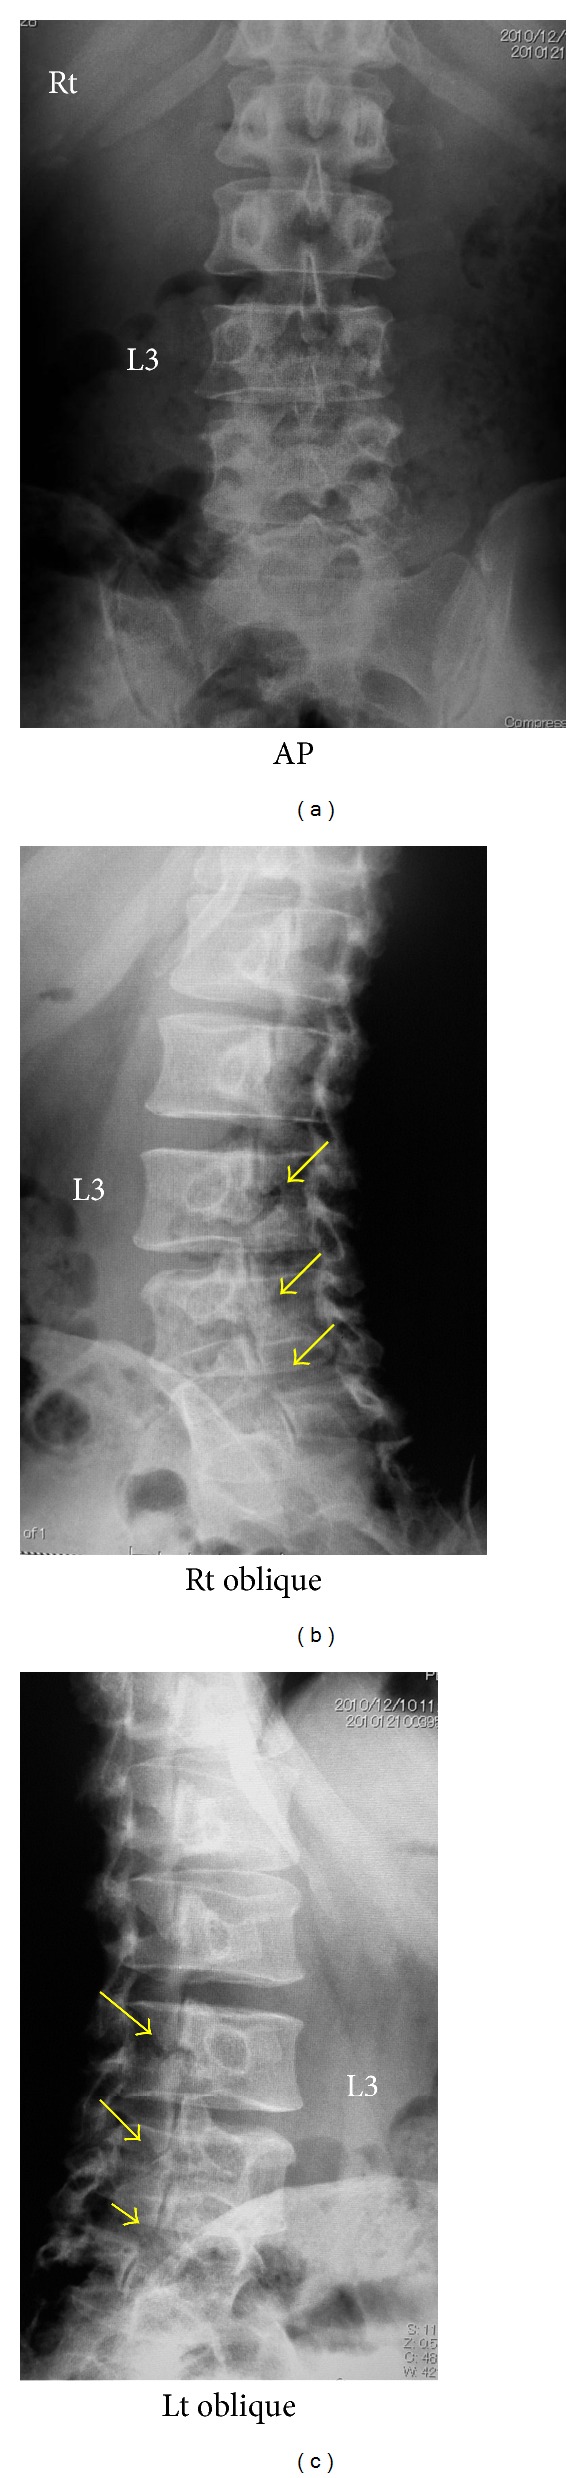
Plain anteroposterior and oblique radiographs at initial presentation show bilateral lumbar spondylolysis at L3–5.
